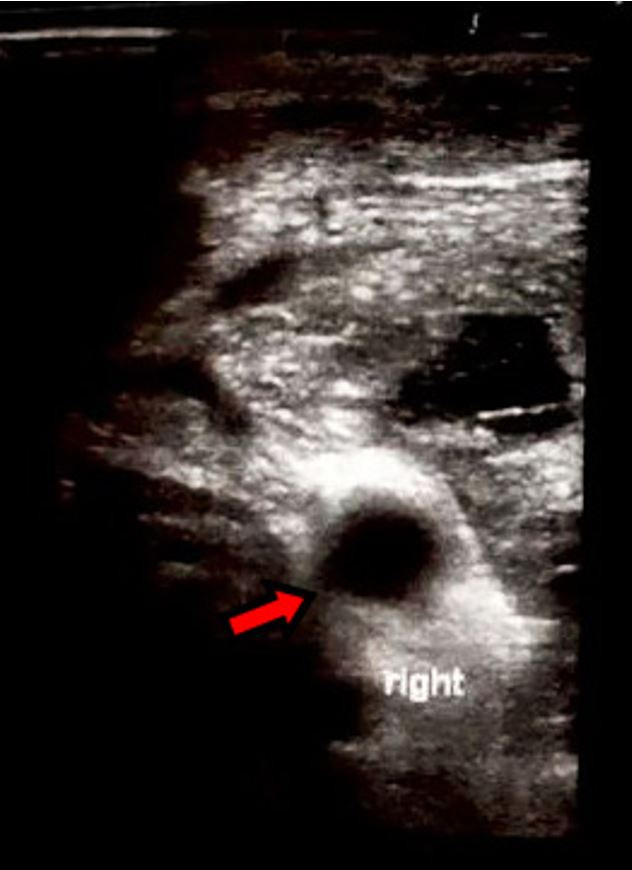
Intraoperative ultrasound revealing a 2 cm heterogeneous right-sided mass consistent with an abnormal right upper parathyroid gland.
